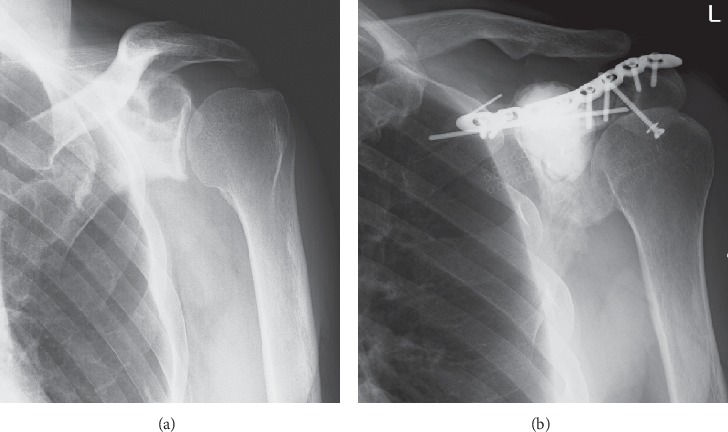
Case #3: 40-year-old male with grade 2 chondrosarcoma of the left scapula. (a) Preoperative X-ray and (b) 4 years after treatment with osteoarticular extracorporeal irradiated autograft.
